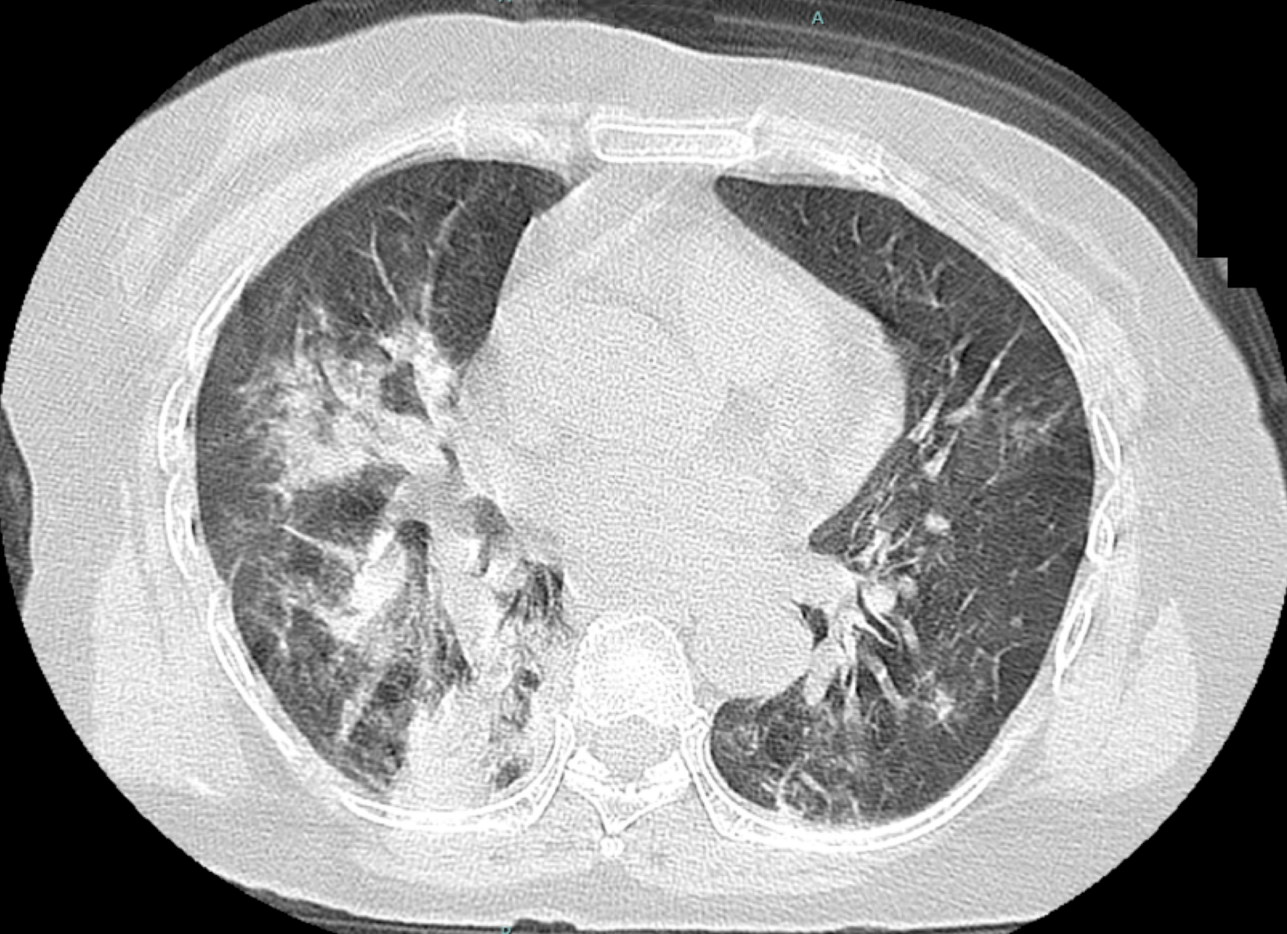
Chest computed tomography of the patient on admission. Chest computed tomography shows consolidation in the right lobe and ground glass opacity in the left lobe.
